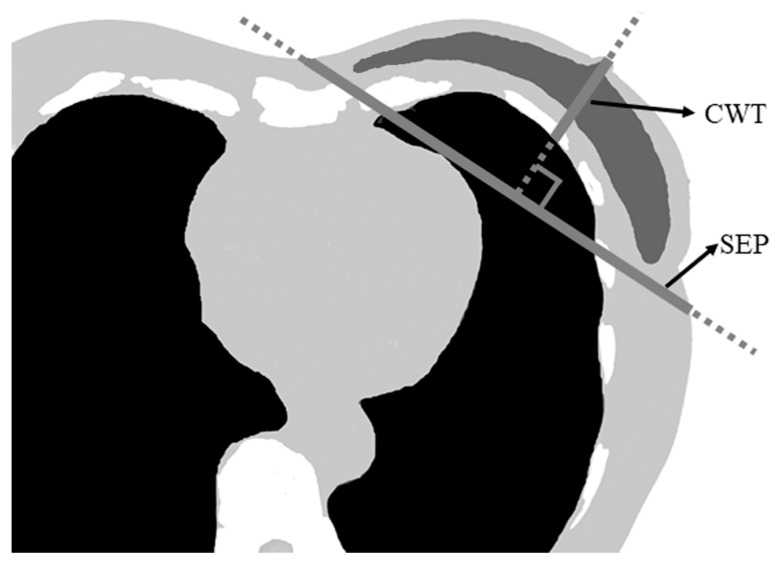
Single-slice CT parameters. Separation was defined as the distance along the posterior edge of the tangent fields at the nipple level. Chest wall thickness was defined as the distance from the nipple surface to the lung on a perpendicular line of breast separation. CWT—chest wall thickness; SEP—breast separation.
